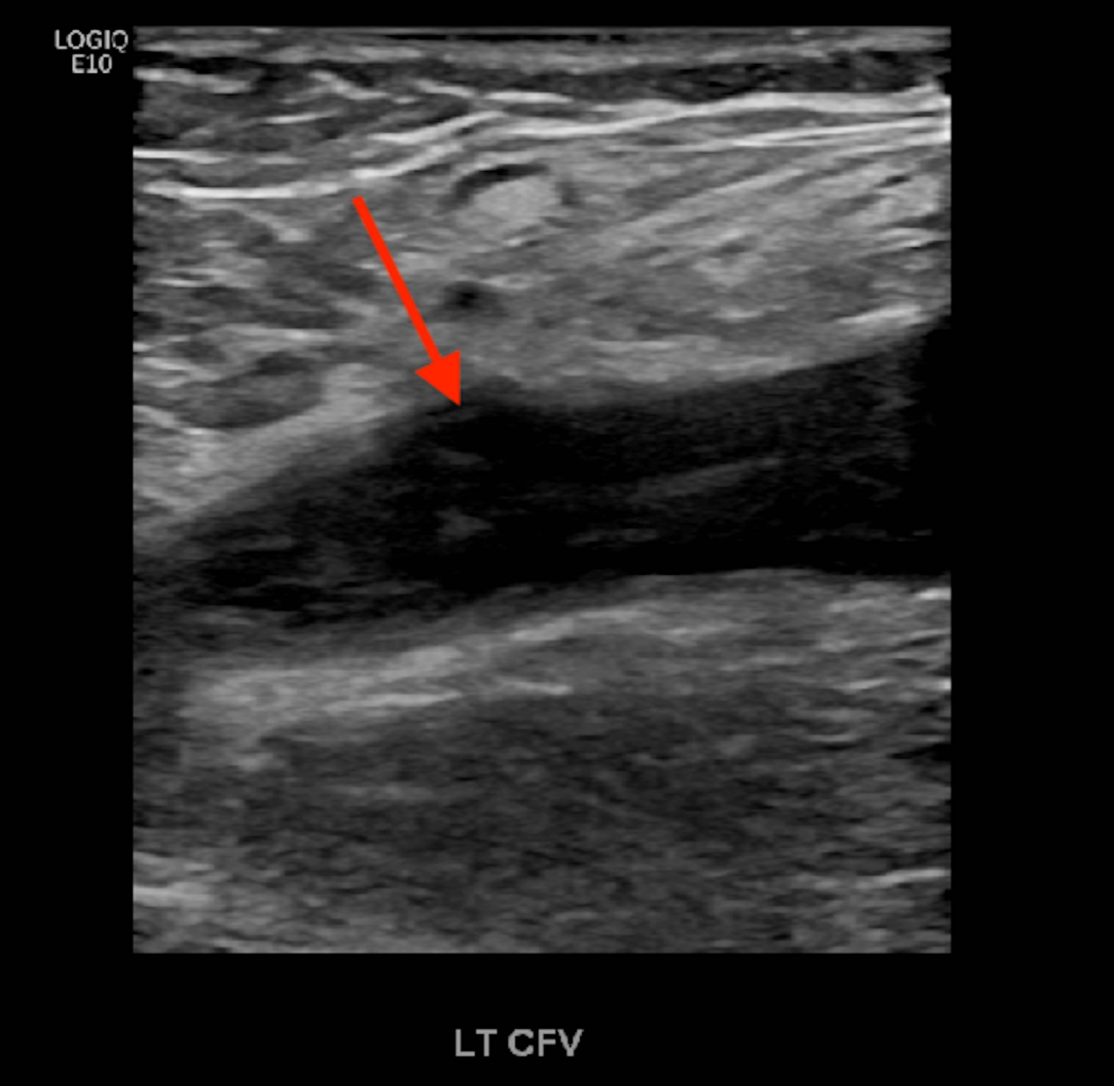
Ultrasound demonstrating echogenic (thrombosed) blood within the common femoral vein (red arrow).
